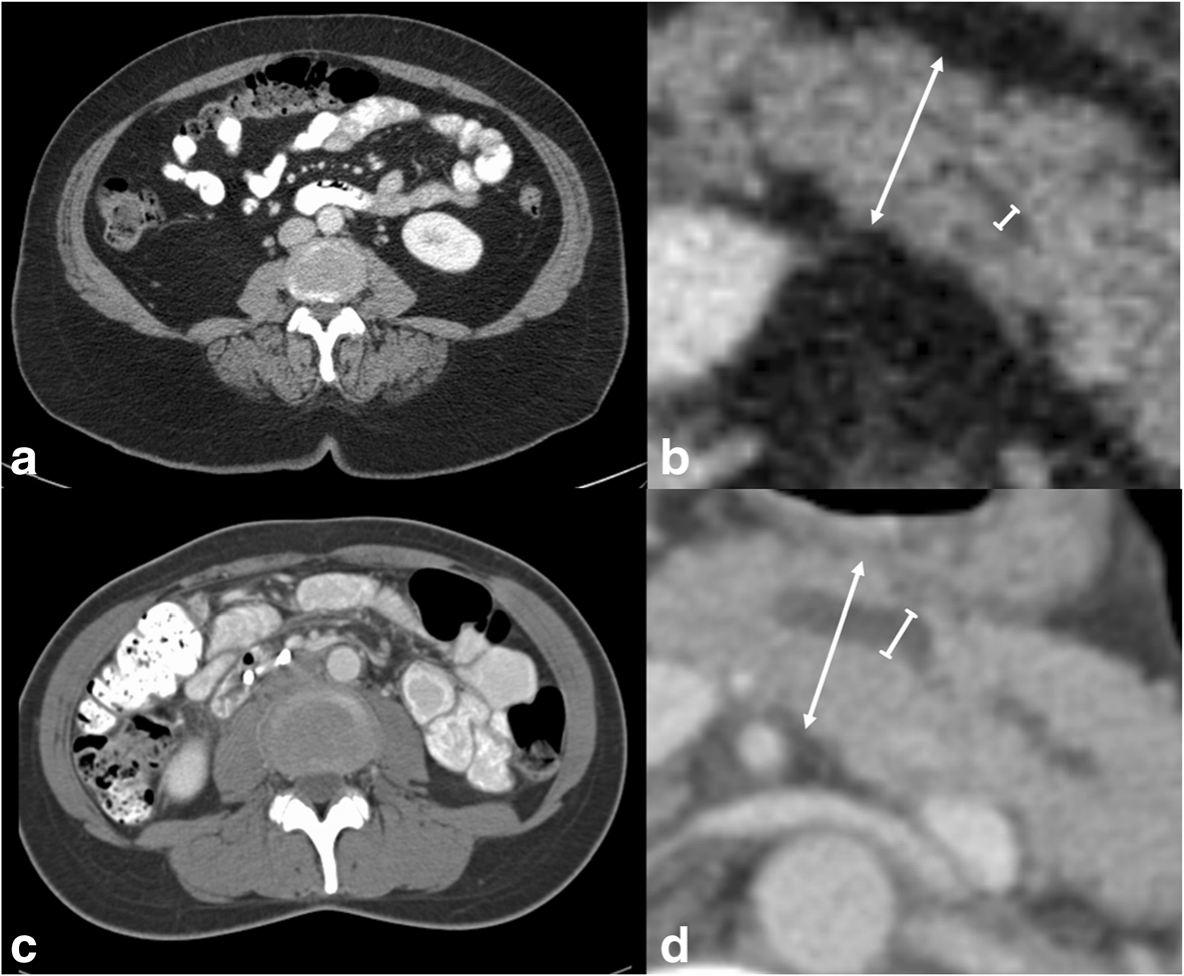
Sample CT images of two female patients with high (a, b) and low (c, d) risk profile for postoperative fistula and complications. a,b. Sample patient with grade C fistula and grade IIIb complications. A_VAT_ (a) was 203.2 cm^2^, DPP was 19.7 mm and DPD was 2.3 mm, respectively (arrow and line in b). c, d. Female patient with no fistula and no complications. A_VAT_ (d) was 26.6 cm^2^, DPP was 18.7 mm and DPD was 4.0 mm, respectively (arrow and line in d)
